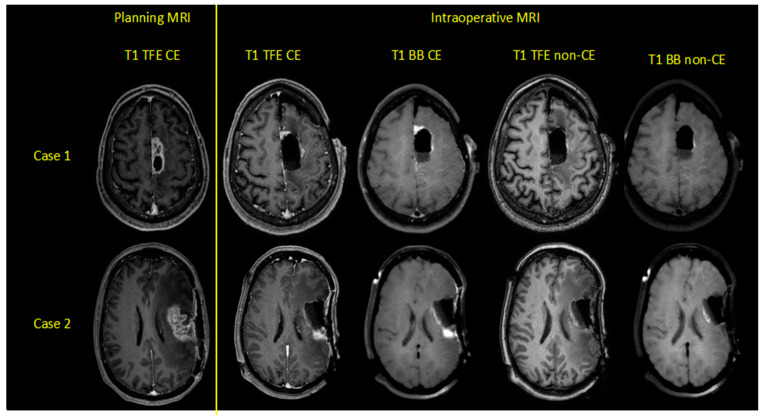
Exemplary cases of two patients harboring left-hemispheric glioblastoma multiforme, depicting contrast-enhanced (CE) and non-CE turbo field-echo (TFE) and Black Blood (BB) sequences for preoperative planning and intraoperative magnetic resonance imaging (iMRI).
